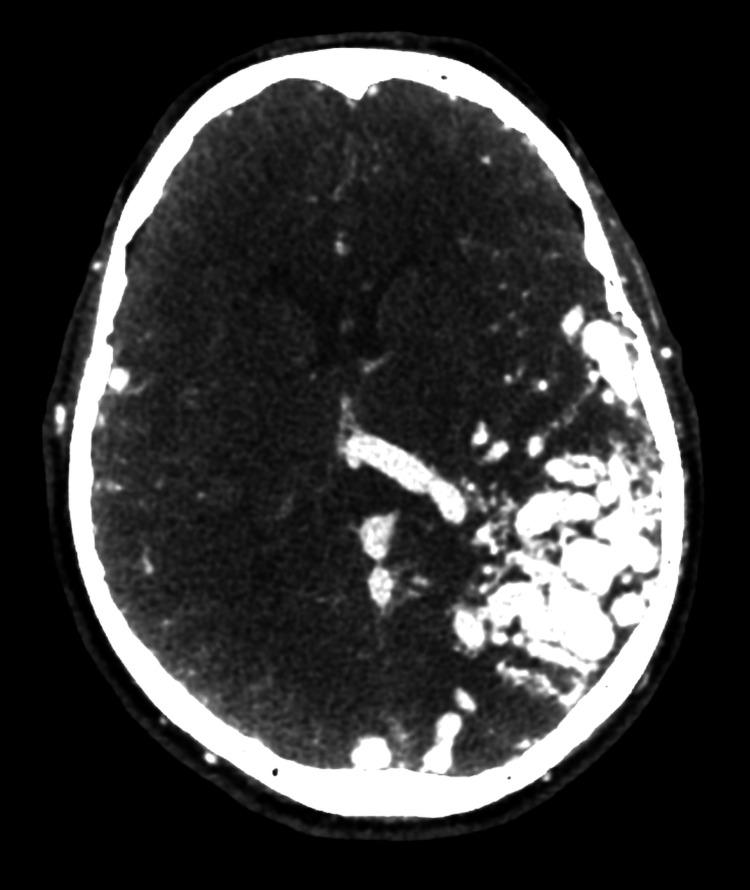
CTA of the giant AVM CT: computed tomography angiography; AVM: arteriovenous malformation Contrast-enhanced CTA revealing a giant left parieto-temporo-occipital AVM
